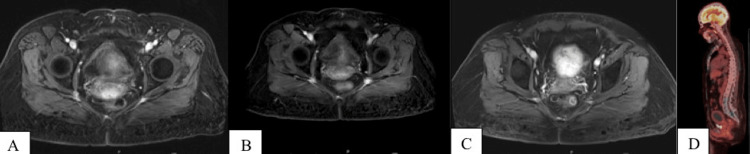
Imaging pre- and postchemoradiation (A) MRI pelvis June 3, 2020: axial T1 with contrast. (B) MRI pelvis September 23, 2020: axial T1 with contrast. (C) MRI pelvis September 22, 2021: axial T1 with contrast. (D) December 3, 2021 positron emission tomography (PET)/CT: sagittal view with avidity in vagina and cervix.
